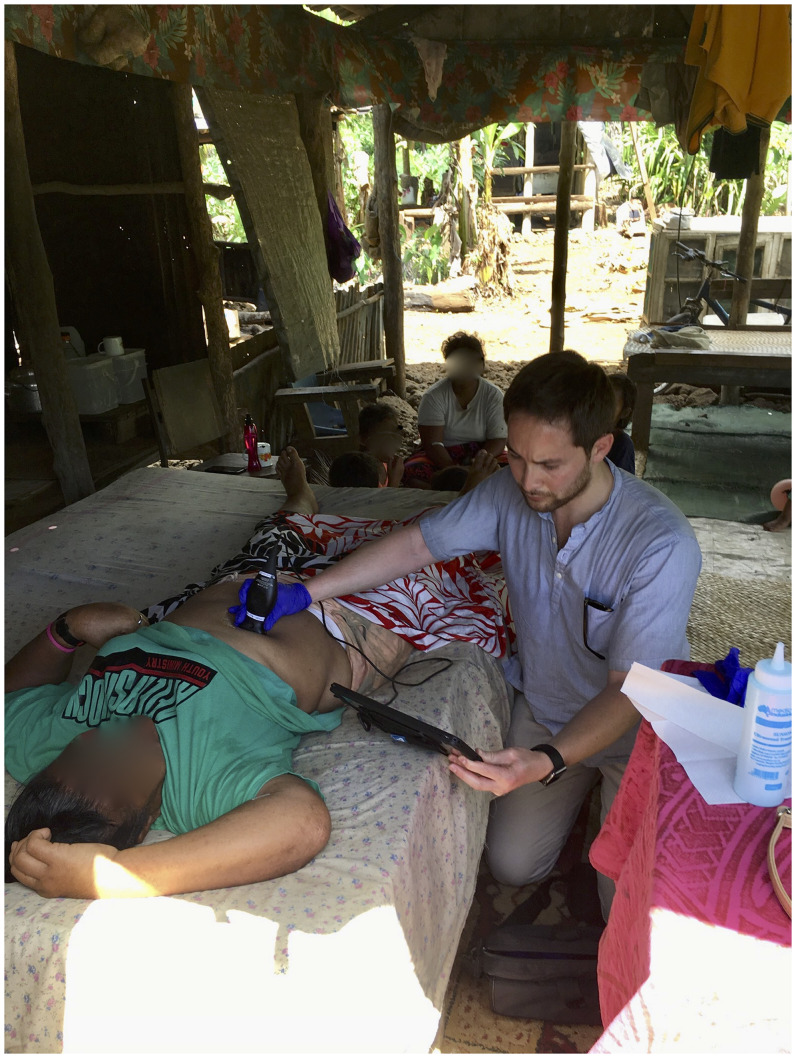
Nonexpert POCUS operator (S.A.H.) performing field examination for gallstones using the Butterfly iQ ultrasound probe. POCUS = point-of-care ultrasound.
